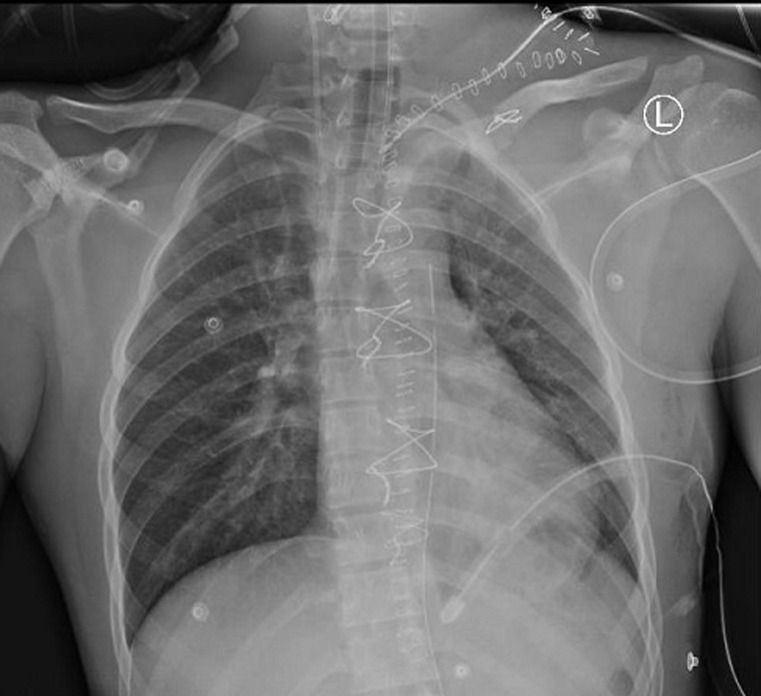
plain chest X-ray, anteroposterior view in the supine position on the first postoperative day
